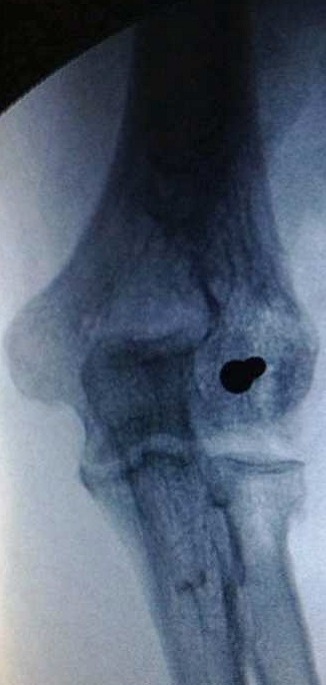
Contrôle radiographique de face du montage
